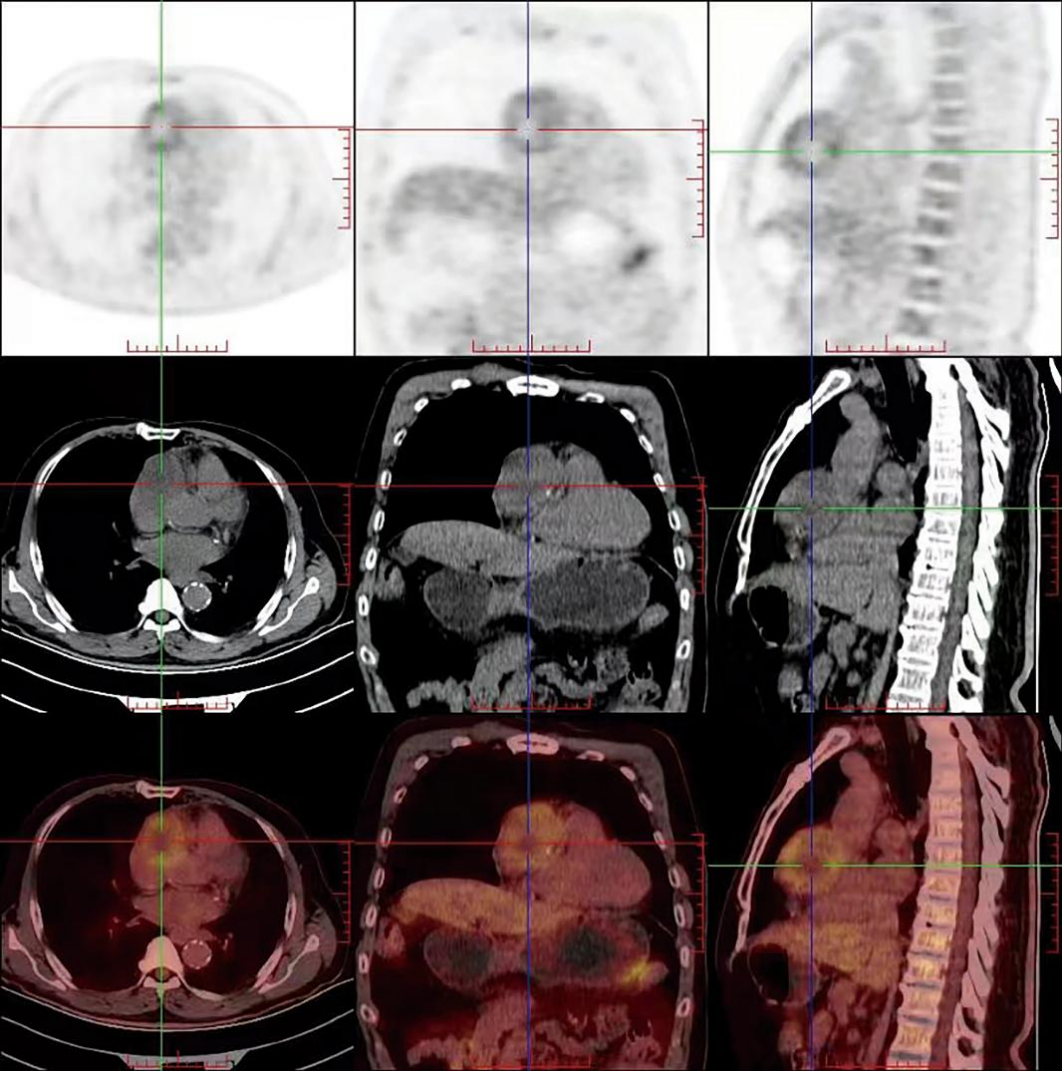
Positron emission tomography-computed tomography showing lesions with uneven ring-shaped increased uptake of fluorodeoxyglucose. The standard uptake value was ∼3.5.
